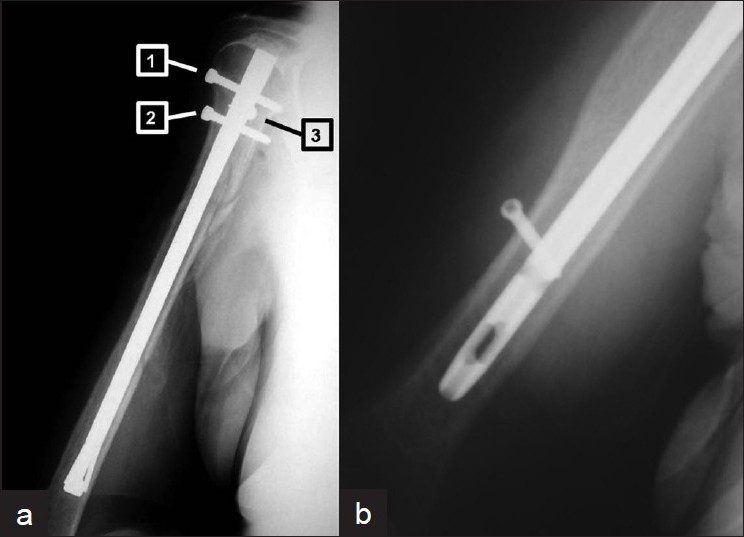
(a) X-ray of the arm including the shoulder joint (anteroposterior view) depicting that proximal locking screws could injure vulnerable soft tissues around the shoulder during antegrade nailing: Screw [1] could damage the circumflex humeral artery, screw [2] could damage the axillary nerve and screw [3] could damage the long head of biceps. (b) X-ray of the lower arm (lateral view) depicting a “miss a hole” situation after the use of a targeting device
